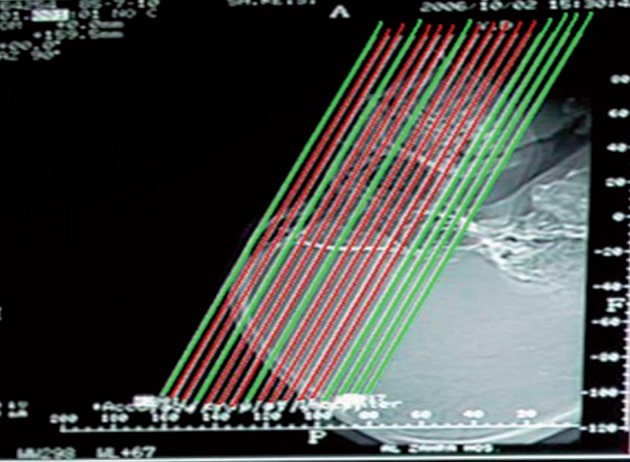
Standard CT protocol; coronal section with 2-3 mm collimation and 5 mm image interval. (Deleted slices is shown in green and limited series is marked as red)
